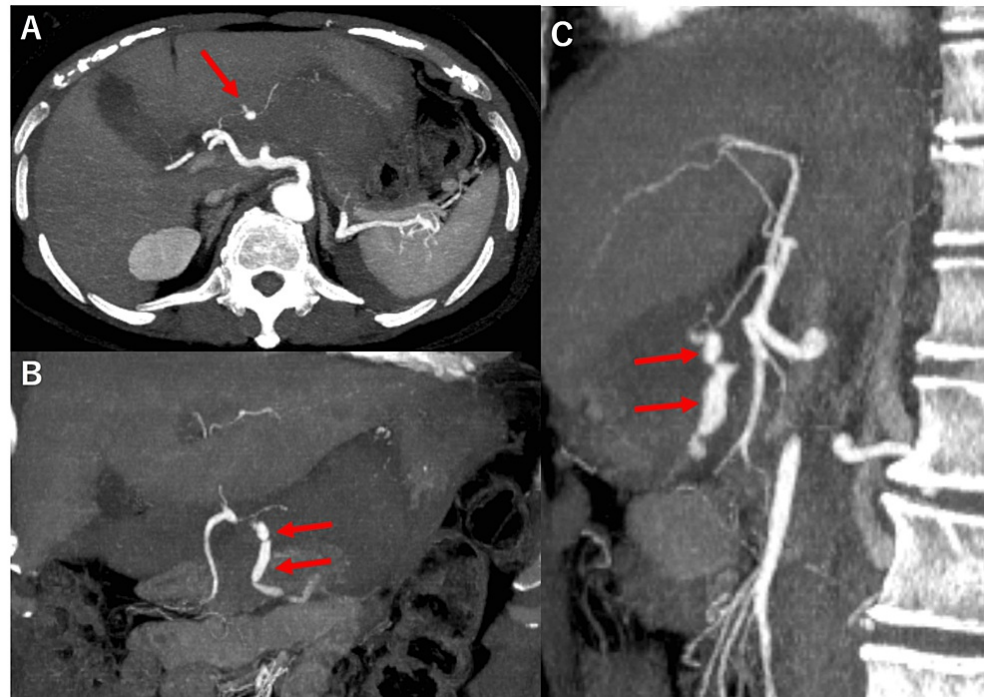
Reconstructed contrast-enhanced computed tomography Reconstructed contrast-enhanced CT was performed at the time of injury, with pane A showing the axial, pane B the coronal, and pane C the sagittal images. Since there was a pseudoaneurysm in the right gastric artery and continuous extravasation (red arrow), it was judged that the right gastric artery was the injured vessel.
